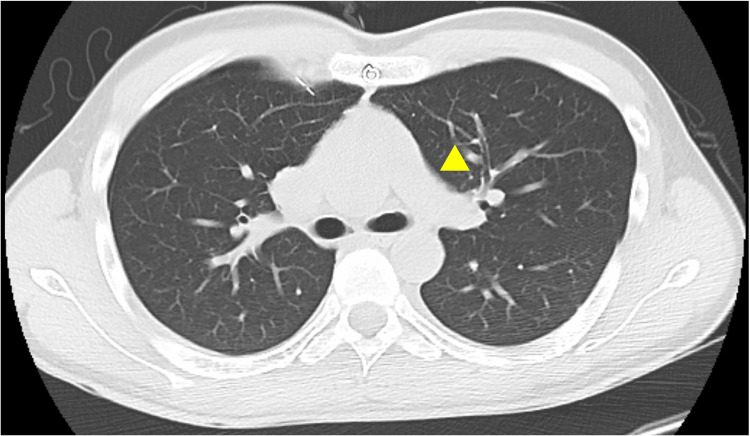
CT of the great arteries The PA lies in front of the aorta, suggesting that the patient had undergone an arterial switch operation using the Lecompte maneuver. Although not contrast-enhanced, no obvious stenosis of the left PA is observed (yellow arrowhead). PA: Pulmonary artery
